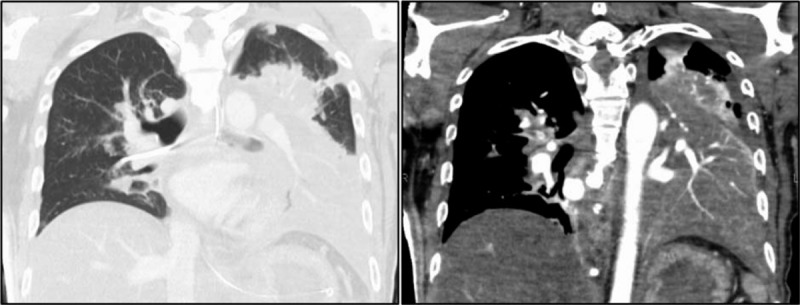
Chest computed tomography images without (left panel) and with (right panel) contrast enhancement. Note the enhanced pulmonary vasculatures within an airless low attenuating intense consolidation in the lower left lobe, known as a computed tomography angiogram sign, and multiple patchy infiltrates in both lobes.
